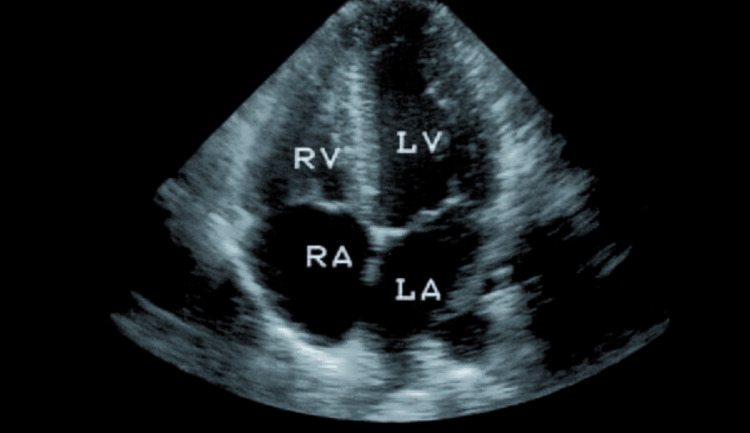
A normal echocardiogram (apical four-chamber view), without any left ventricular dysfunction or regional wall motion abnormality RA: right atria; LA: left atria; RV: right ventricle; LV: left ventricle
